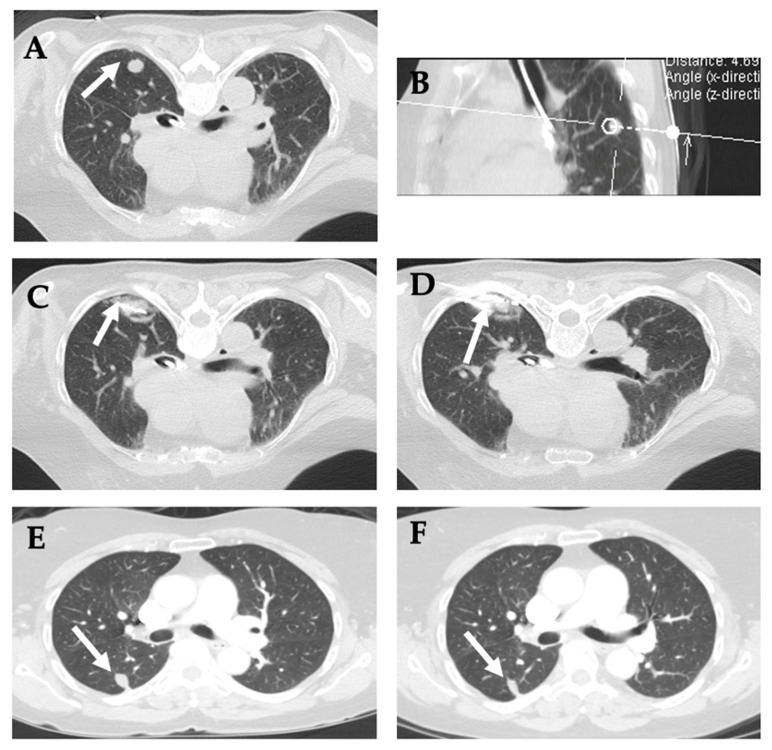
Cryoablation of right lower lobe lung metastatic lesion suspected for colorectal cancer metastasis. (A,B) CT images of the chest show the 1.2 cm nodule (white arrow) in the right lower lobe of the lung. (C) Under CT image-guidance, two Endocare PCS-17R cryoablation probes were advanced via a posterior approach, which bracketed the tumor to optimize the ablation coverage of the lesion. (D) Under CT image-guidance, a 19-gauge needle was advanced down to the lesion. An image was obtained and placed into the medical record. Samples were obtained for evaluation. Cryoablation was performed with three freeze cycles that lasted three, seven, and ten minutes, respectively, interposed with three minutes passive thaw cycles. (E) 6 and (F) 12-month follow up images show shrinking scar.
